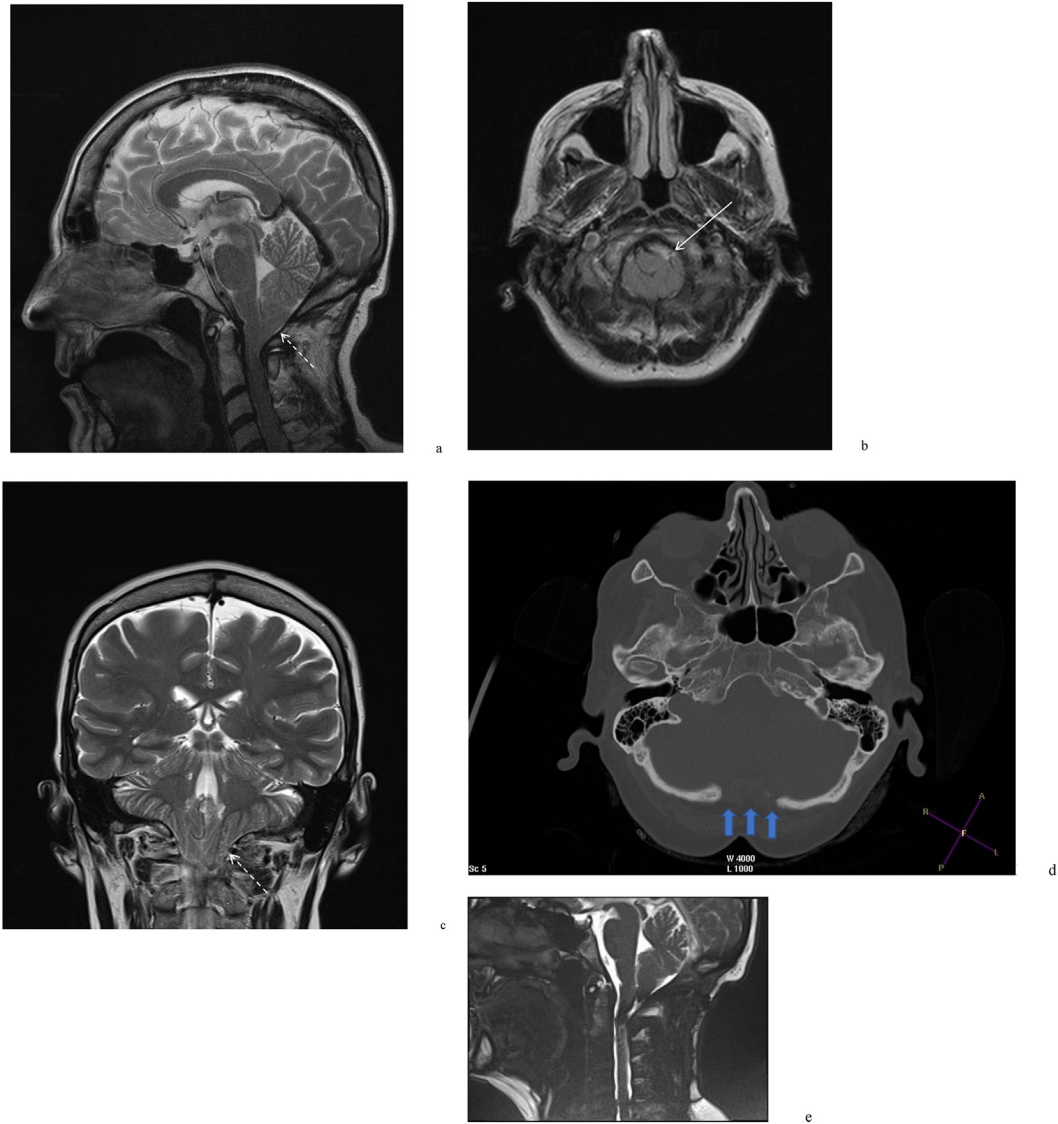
Sagittal (a), axial (b) and coronal (c) T2 weighted images from a brain MRI demonstrating crowding of the CSF spaces at the level of the foramen magnum (solid white arrow) and a peg-like deformity of the cerebellar tonsils (white dashed arrow). A decompressive posterior fossa craniectomy (blue arrows) was performed (d) with CSF flow voids along the craniocervical junction anteriorly and posteriorly which appear as areas of darker signal (e). (For interpretation of the references to colour in this figure legend, the reader is referred to the Web version of this article.)
